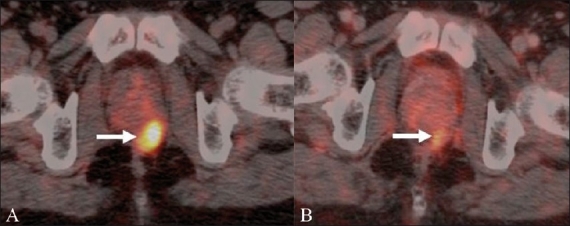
A 52-year-old man with metastatic CRC imaged with FDG PET / CT before and after targeted therapy. Fusion PET / CT study (A) prior to targeted therapy shows an FDG-avid metastatic retroprostatic nodule (arrow). There is significant reduction in the metabolism and size of the recurrent nodule (arrow) on the post-therapy follow-up PET / CT image (B), suggesting therapeutic response
